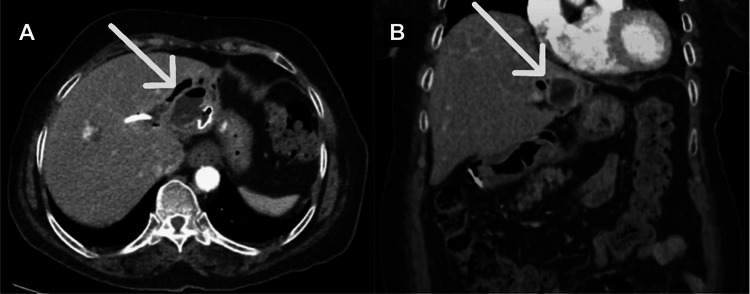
Axial (A) and coronal (B) computed tomography scans of the abdomen showing liver abscesses due to hepatolithiasis (arrows)
